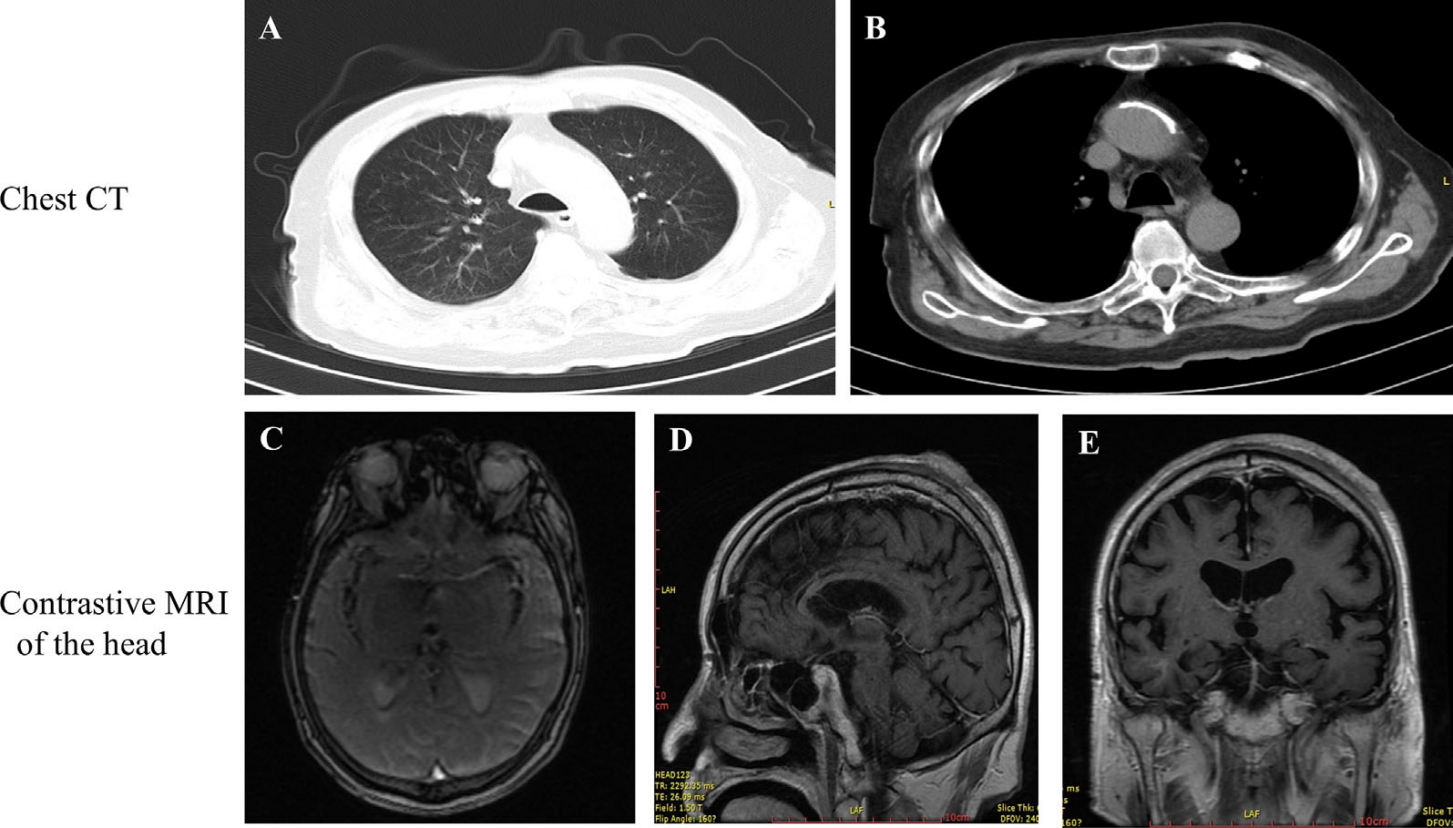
(A, B) Examination of chest CT. (C) superior view, (D) lateral view and (E) posterior view of the scull during contrast MRI after 10 months treatment with anlotinib.
